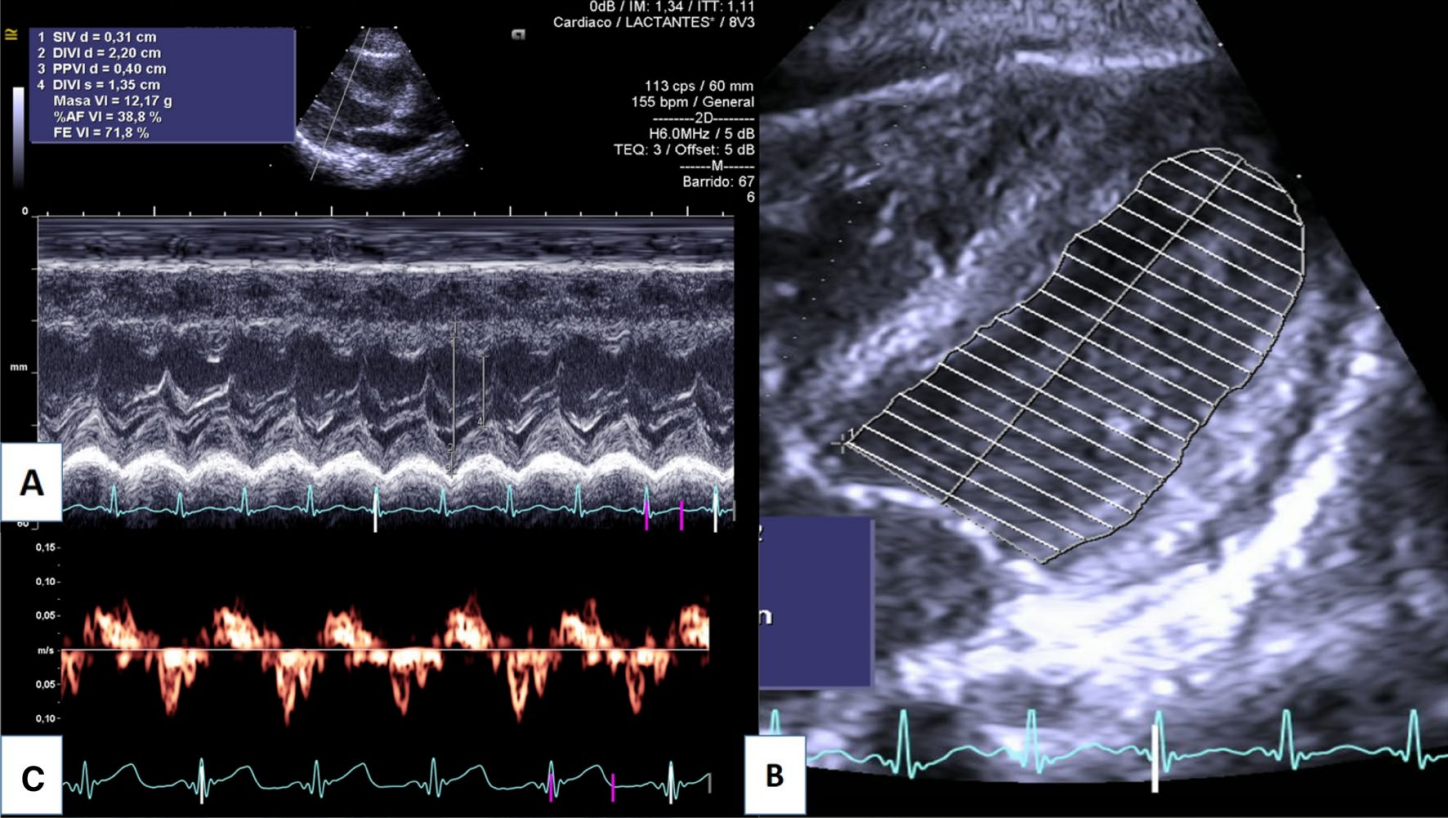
2D Echocardiography. A IVSd, interventricular septum thickness at end-diastole; LVIDd, left ventricular internal dimension at end-diastole; LVIDs, left ventricular internal dimension at end-systole; PWD, posterior wall at diastole; LVM, Left ventricular mass. B LVEF, left ventricular ejection fraction using biplane Simpson´s method. C Left ventricular tissue doppler velocities (s´,e´,a’) of the lateral side of the mitral annulus
